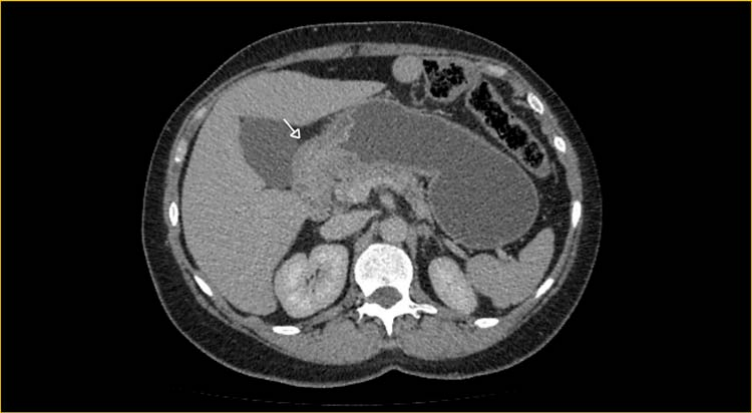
Portal phase transversal section image on CT showing excluded stomach distention with parietal thickening of the pylorus and antrum (arrow).
